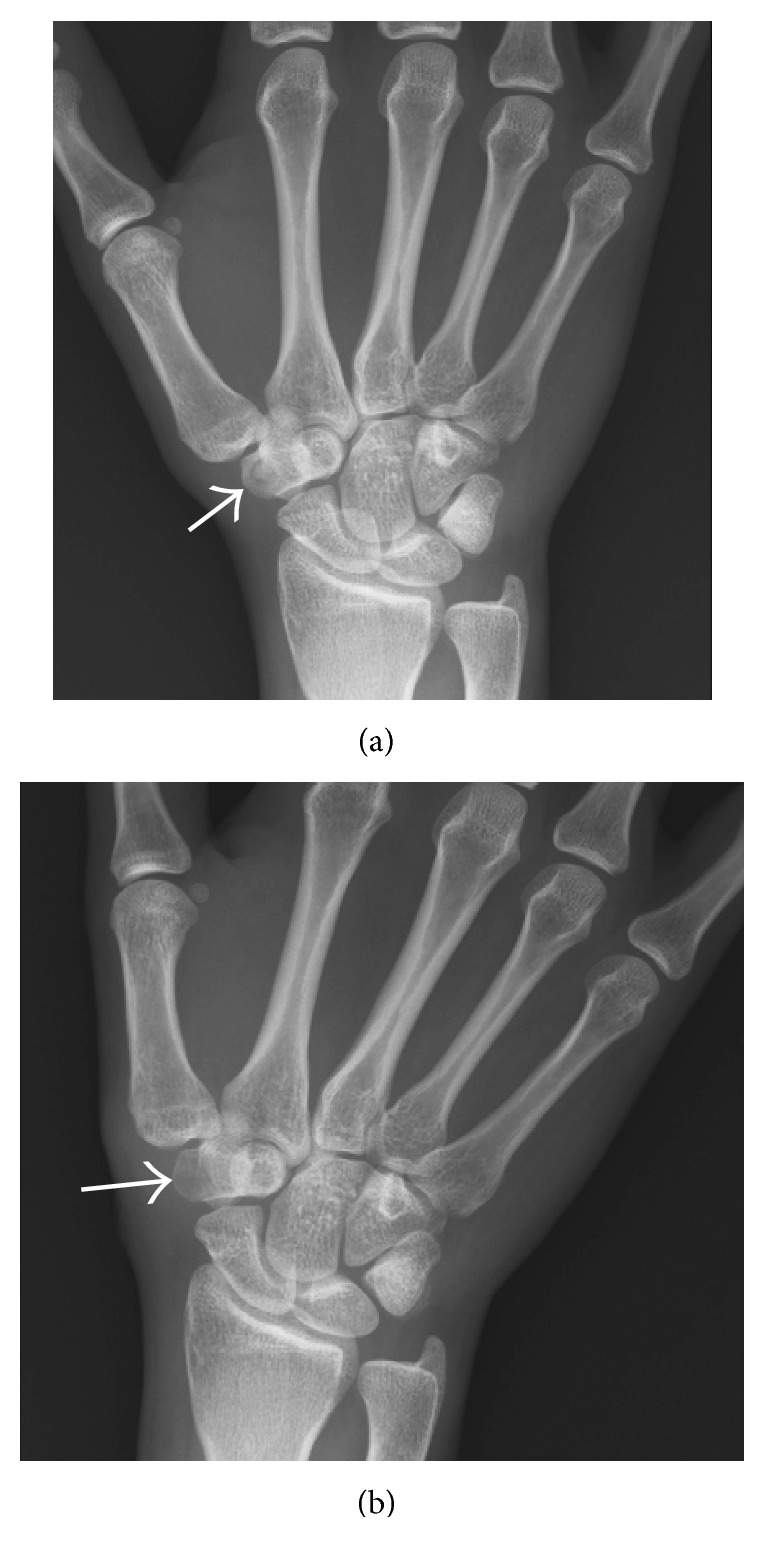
(a) Preoperative radiography of the wrist showing nidus (arrow). (b) Postoperative radiography of wrist.
